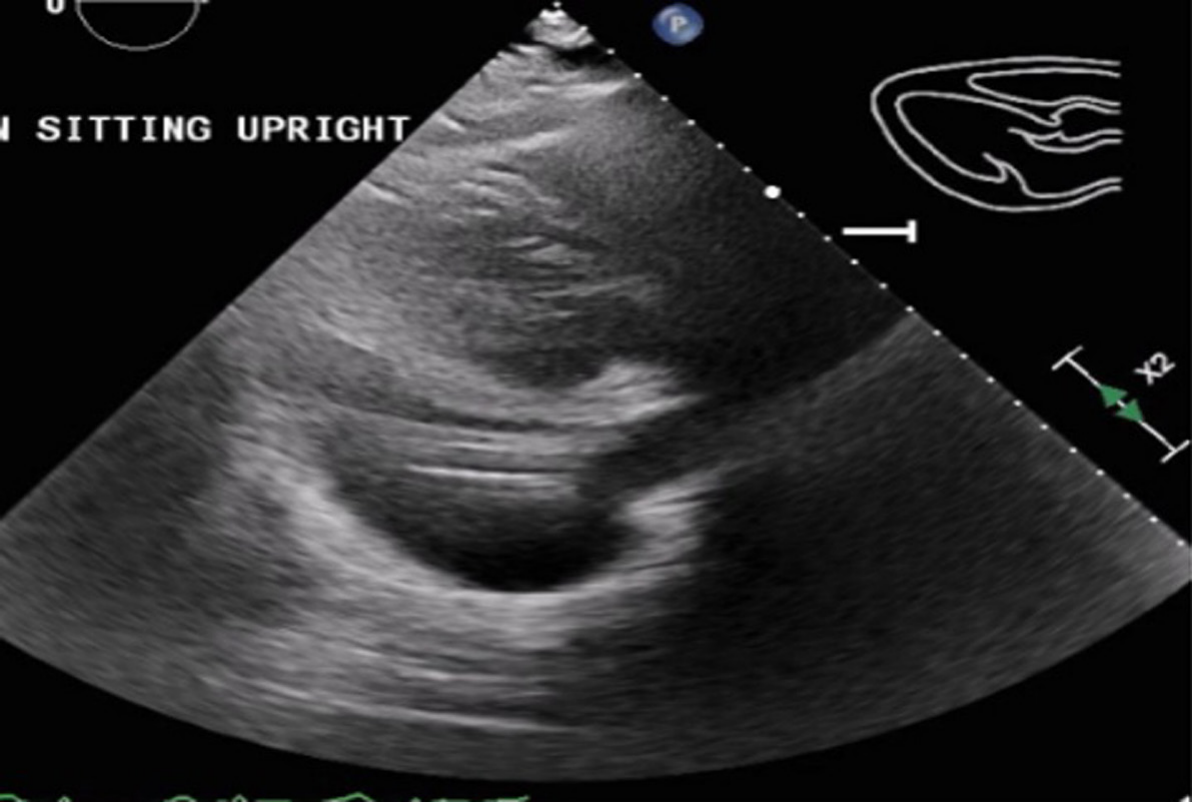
Echocardiogram revealing near complete resolution of pericardial effusion.
